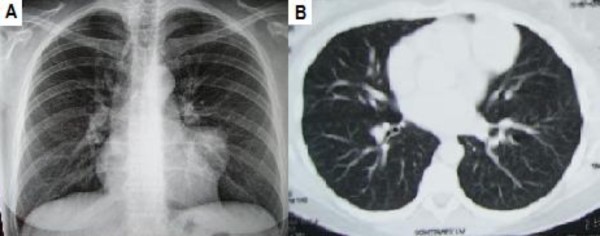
(a) Pre-operative chest radiography. (b) Pre-operative CT scan.
